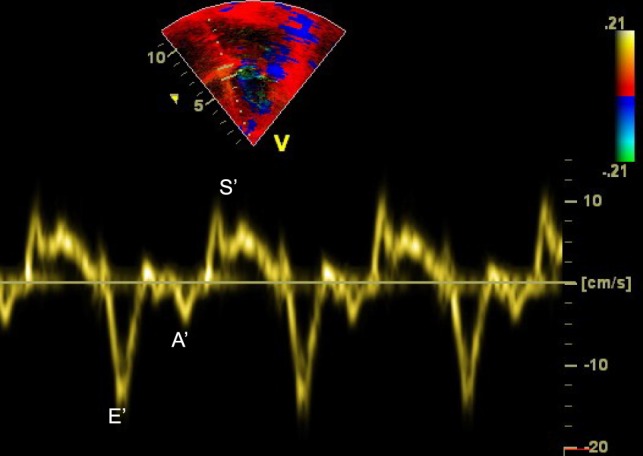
Tissue Doppler imaging (TDI) at the level of the interventricular septum from apical four-chamber view demonstrating the *E*′, *A*′, and *S*′ waves. *A*′, late diastolic ventricular filling during atrial contraction wave; *E*′, early ventricular filling wave; *S*′, systolic wave.
